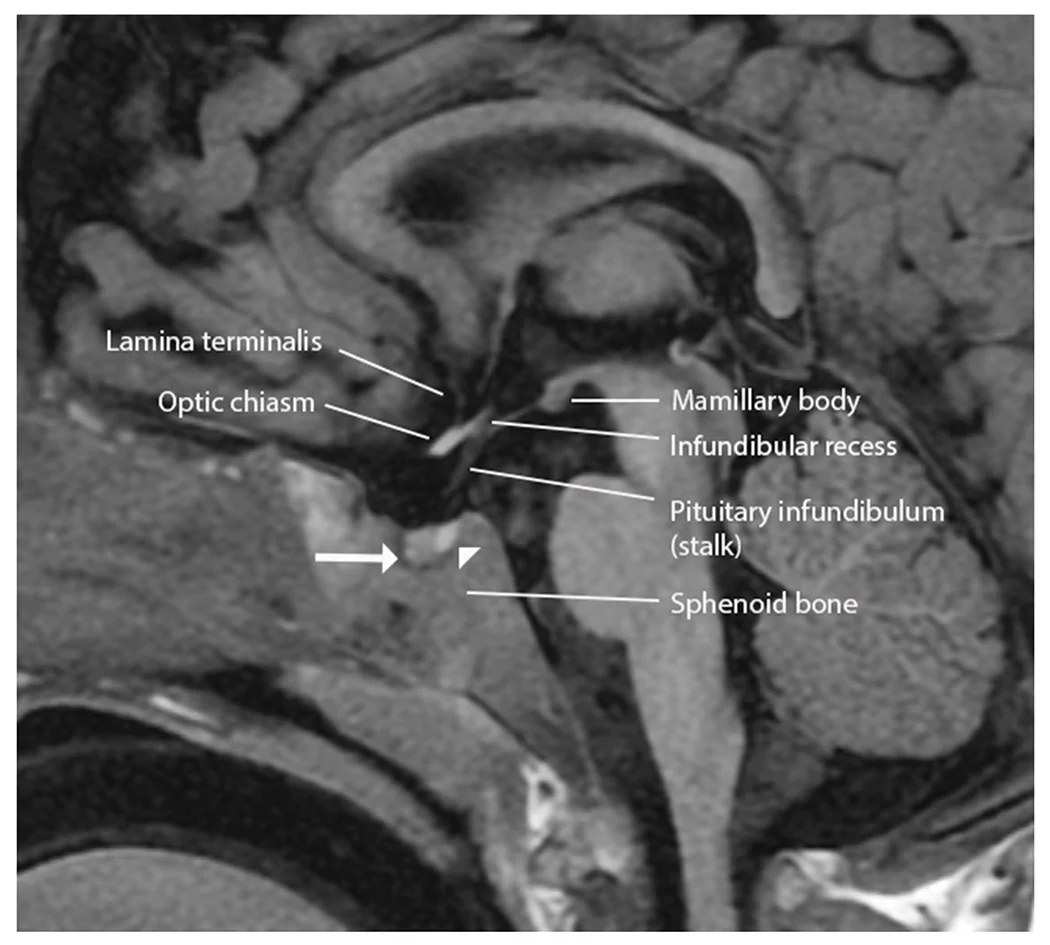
Normal appearance of adenohypophysis in a 6-month-old male infant with seizure (no endocrinopathy). Sagittal T1-weighted brain magnetic resonance imaging focused on the pituitary gland demonstrates an isointense adenohypophysis (*arrow*) distinguishable from the hyperintense posterior pituitary bright spot (*arrowhead*). Additional anatomic structures of the hypothalamic region are labeled
